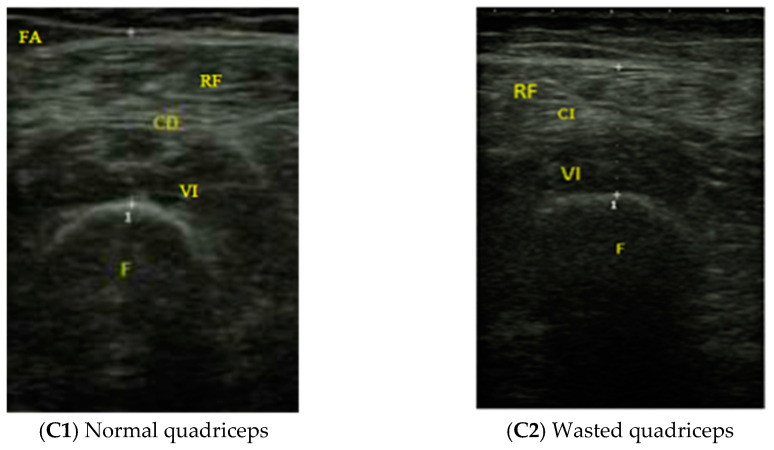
Ultrasound images. (A) Masseter muscle (A1 normal and A2 wasted). A1 (normal masseter muscle), A2 (wasted masseter muscle), (M: masseter muscle; MD: right jaw; MI left jaw); (B) Biceps brachii (B1 normal and B2 wasted). B1 (normal bíceps brachii), B2 (wasted bíceps brachii), (H: humerus; B: Biceps); (C) Quadriceps femoris (C1 normal C2 wasted). C1(normal quadriceps RF-VI), C2 (wasted quadriceps RF-VI), (FA: subcutaneous celular tissue; RF: rectus femoris; VI: vastus intermedius; F: femoris CD: right quadriceps humerus; B: Biceps).
